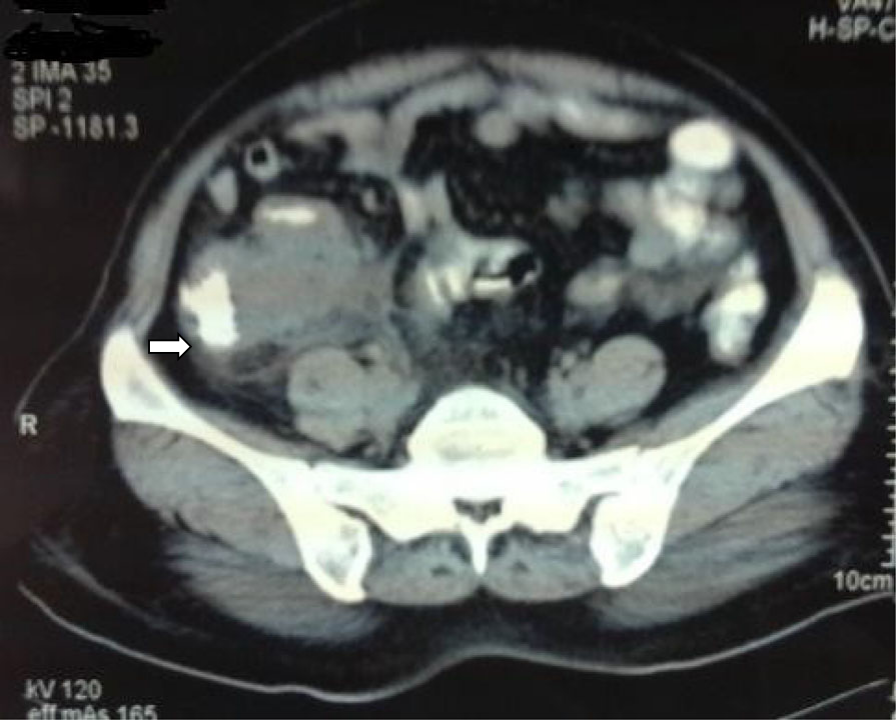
CT scan showing appendicular lump. *Arrow* showed an ill-defined hypo-dense peripherally enhancing collection about 4 × 3.65 cm in the right iliac fossa. Few air locules with asymmetric mural thickening of ileum, caecum, and ileocaecal junction
